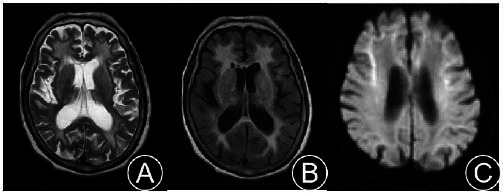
Cranial MRI: Symmetrical large patchy T2WI and FLAIR high signals in the corticomedullary junction, centrum semiovale, and corpus callosum of both cerebral hemispheres (A, B); DWI shows band-like high signals in the corticomedullary junction of both cerebral hemispheres, with high signals in the genu and splenium of the corpus callosum (C).
